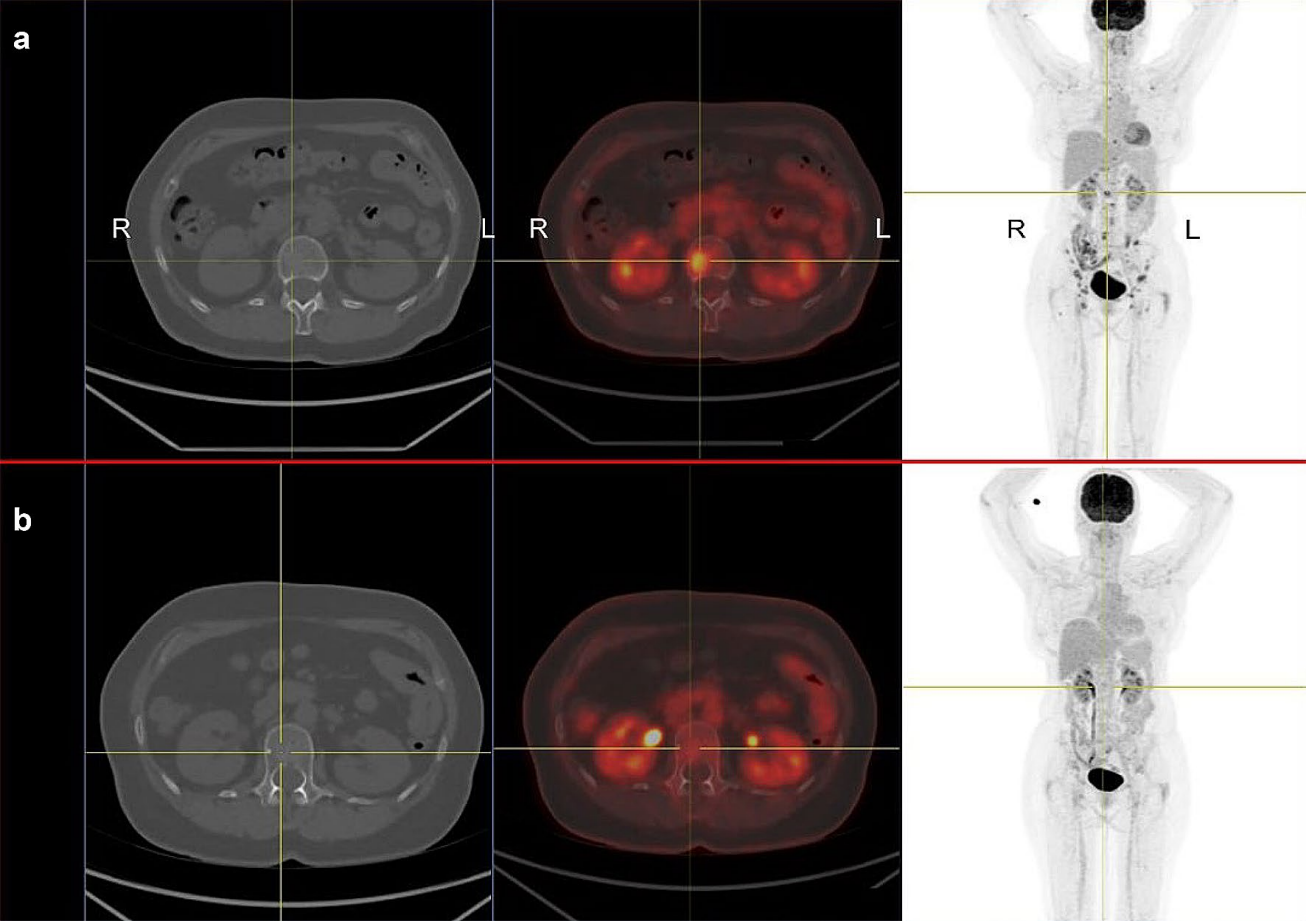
Relapsing metastatic melanoma and response to anti-PD1 treatment in a patient with fingolimod. a 18F-FDG PET/CT scan shows multiple bone metastases of melanoma, including the spine (centered by yellow crosshair). The patient has relapsing-remittent multiple sclerosis (RRMS) and is under treatment with fingolimod. *R* right, *L* left. b After ceasing fingolimod and starting treatment with pembrolizumab the follow-up PET/CT scan after 12 weeks shows a partial remission of all bone metastases including the spine. To date the patient showed no flares of RRMS, despite complete cessation of fingolimod
